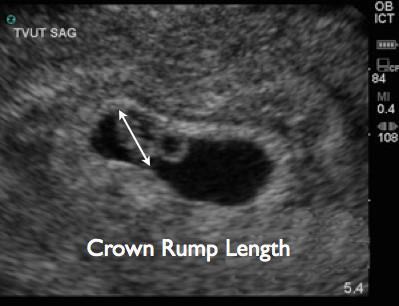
Trans-vaginal ultrasound image demonstrating crown-rump length measurement.
